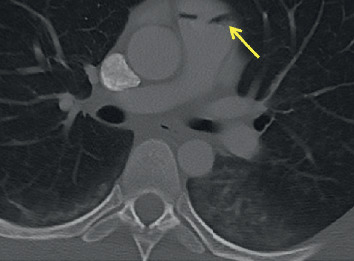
Axial view of CT chest demonstrating air trapped beneath the pulmonary valves (arrow).
